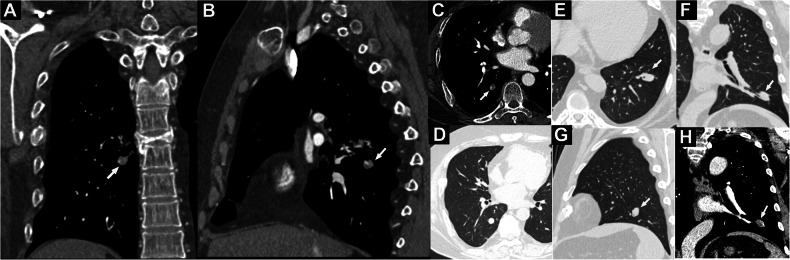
Representative cases of overlying vessel sign and airway involvement. A–D A 29-year-old man with a typical carcinoid tumor and associated ectopic Cushing’s syndrome. A Coronal, (B) sagittal, and (C) axial contrast-enhanced CT showing a peripheral solid nodule in the right lower lobe (RLL) with overlying vessel sign (arrow). D Axial lung window CT showing a solid nodule with a clear boundary in the RLL. E–H A 21-year-old man with a typical carcinoid tumor and associated ectopic Cushing’s syndrome. E Axial, (F) coronal, and (G) sagittal 1 mm thin-slice CT at the lung window showing a peripheral solid nodule in the left lower lobe (LLL) and obstructed adjacent subsegmental bronchus (arrow). H Contrast-enhanced coronal CT at the mediastinal window showing a solid nodule in the LLL with a clear boundary, inhomogeneous enhancement, and overlying vessel sign (arrow)
